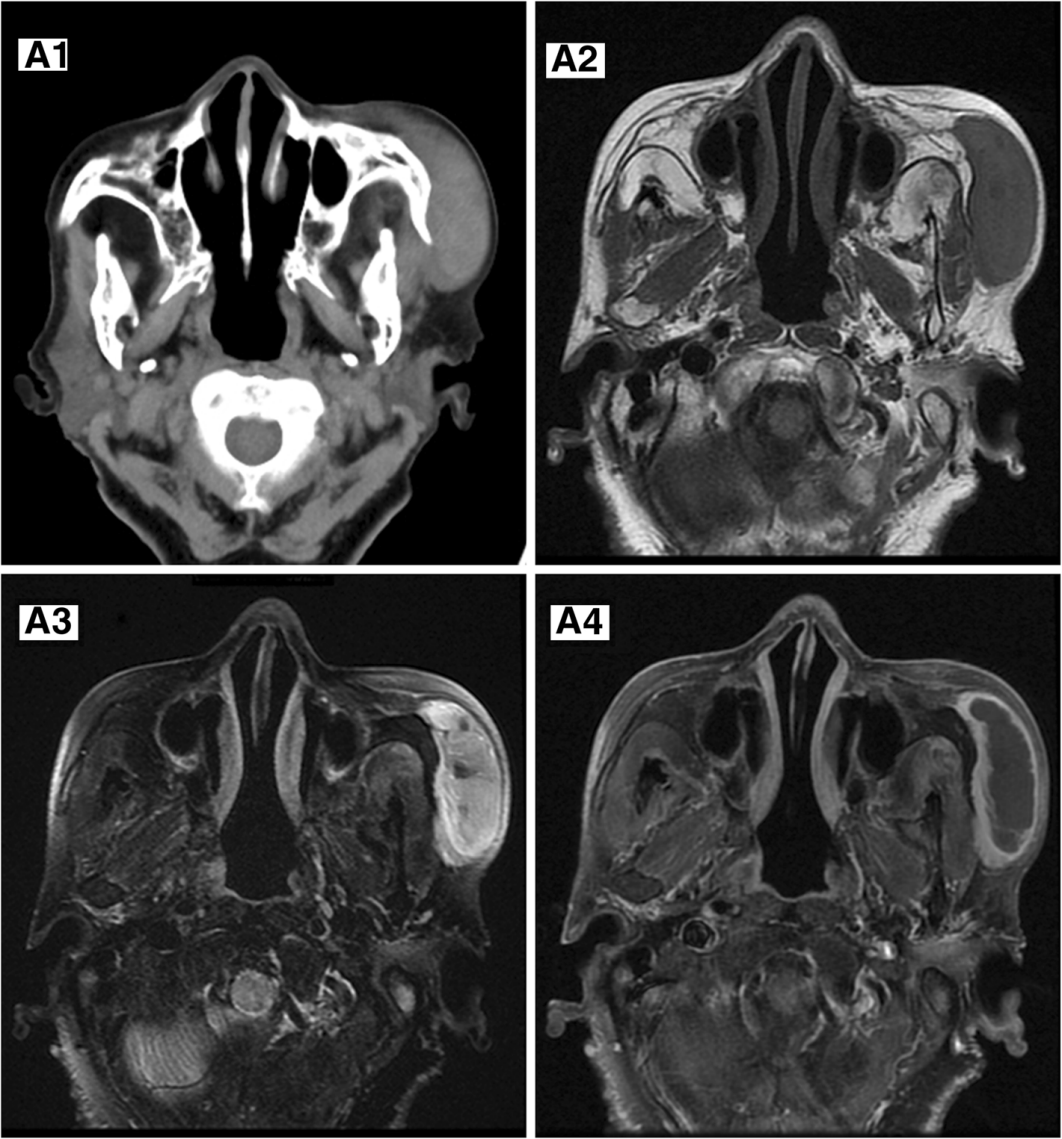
MPNST in a 65-year-old female. Plain CT scan (A1) shows a slightly hyper-attenuated lesion in the left cheek with slight zygomatic arch erosion. On MR scan, the tumor demonstrates hypo-intensity on axial T1-weighted (A2) and heterogeneous hyper-intensity on axial T2-weighted (A3) images. Gadolinium-enhanced T1-weighted images (A4) reveal peripheral enhancement. Note the central hyper-attenuated foci on plain CT and mixed-intense signal on T2WI, which is likely to be hemorrhage. Hemorrhagic cyst was conformed in surgery
